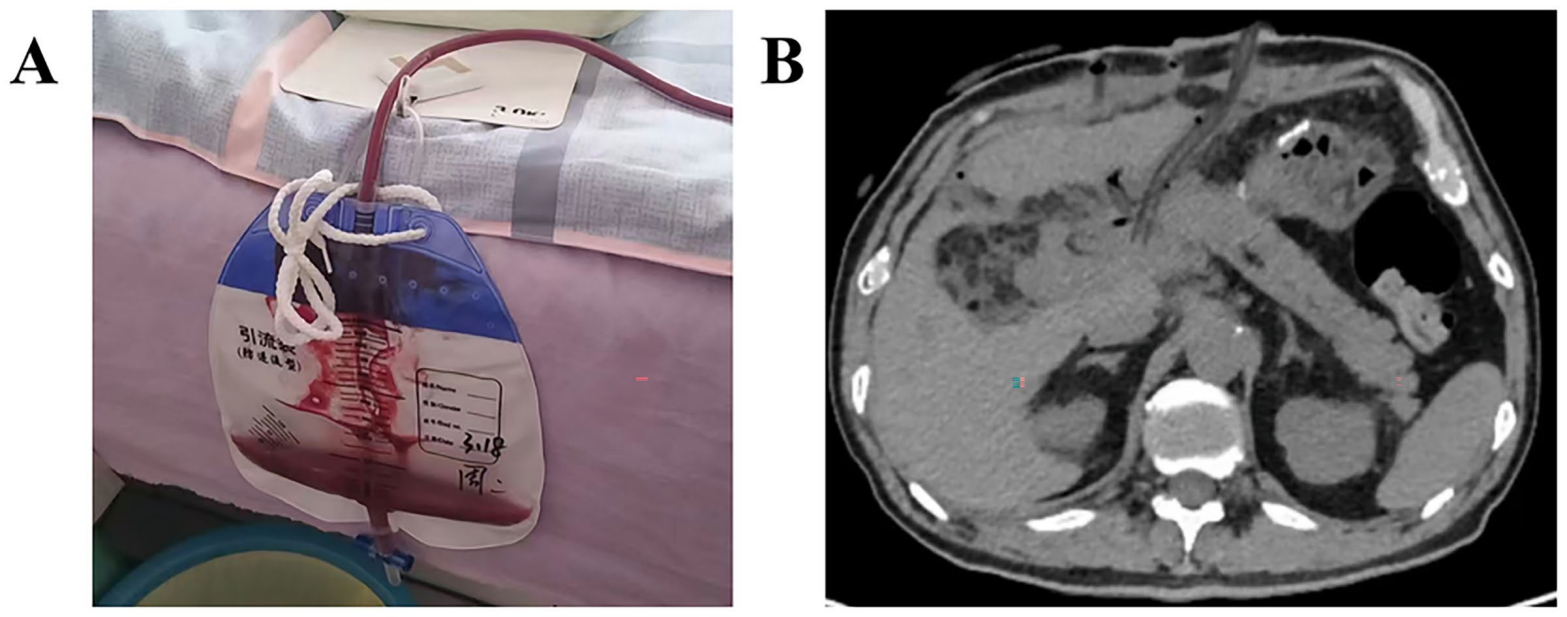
(A) The patient remained hemodynamically stable; a sizeable accumulation of bloody fluid in the drainage bag raised our suspicion of sentinel bleeding. (B) CT showing drainage tubes placed for POPF management to prevent further vascular erosion after emergency laparotomy for initial PPH.
